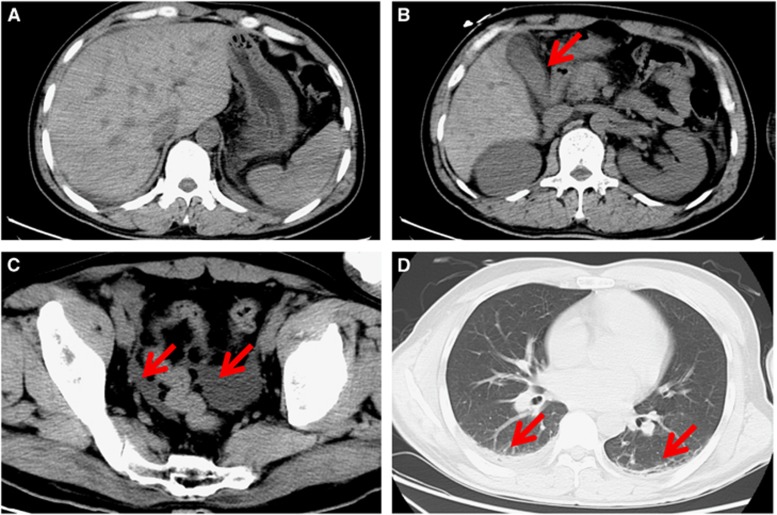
Computed tomography images of the patient on day 7 after clinical presentation of infection. (A) Normal morphology and density of the liver, spleen, pancreas and kidney. (B) Gallbladder wall thickening (red arrow). (C) Fluid in the lower abdominal and pelvic cavities (red arrows). (D) A reduced amount of lower pulmonary effusion and pleural effusion (red arrows).
